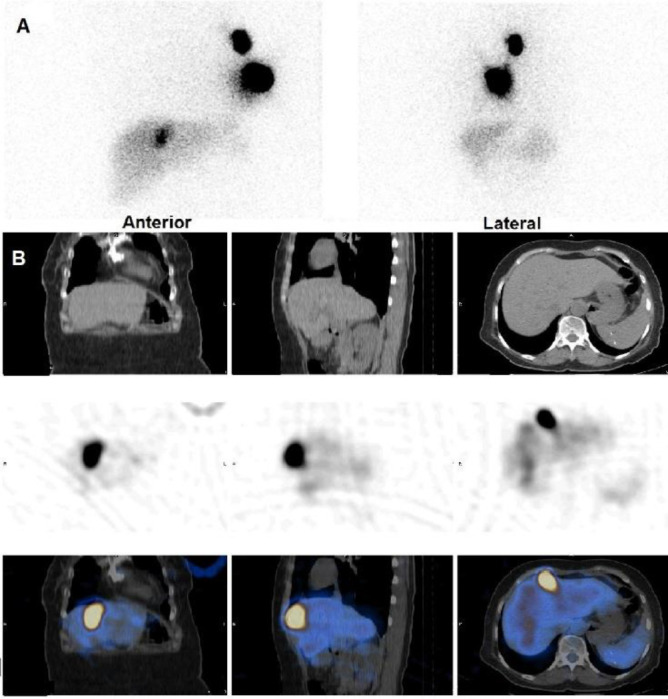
The static images show a focus of increased tracer activity in the left axillary region as well as another focus of increased tracer activity in the zone of liver. B. The SPECT/CT images confine the focus of increased tracer activity in the hepatic region to the falciform ligament with no underlying lymph node
